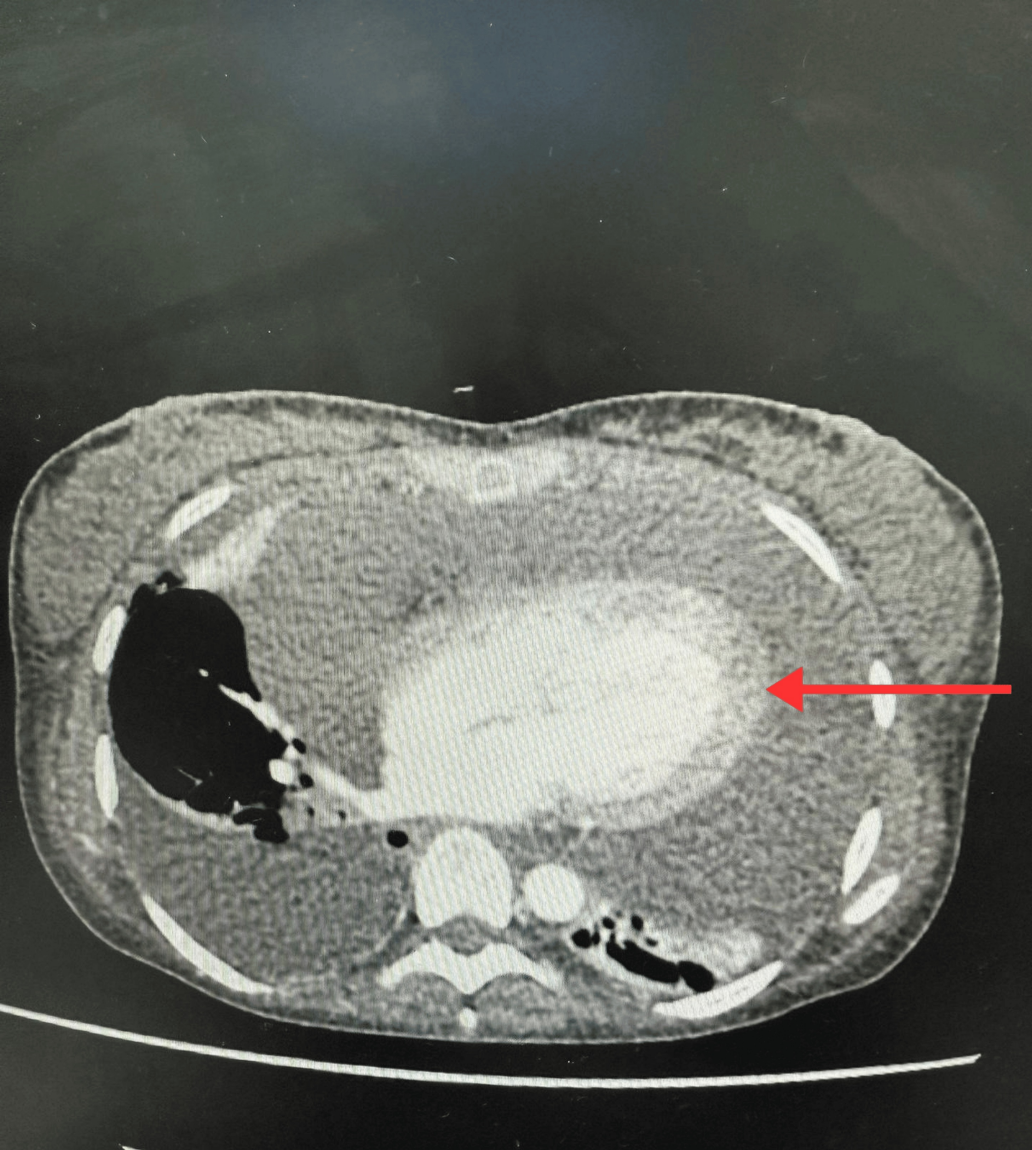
A cross-sectional image of CT chest-abdo-pelvis showing marked pericardial effusion (Red arrow).
